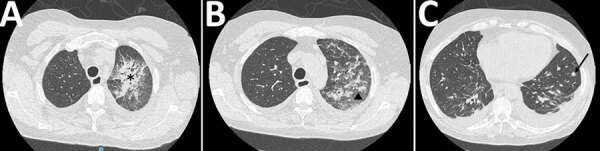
Thoracic computed tomography scans from patients included in a multicenter retrospective study of invasive fusariosis in intensive care units, France, showing findings of fusariosis-related pneumonia. A) Unilateral consolidations (asterisk); B) ground glass opacities (triangle); C) 7-mm nodule (arrow) and bilateral pleural effusion.
